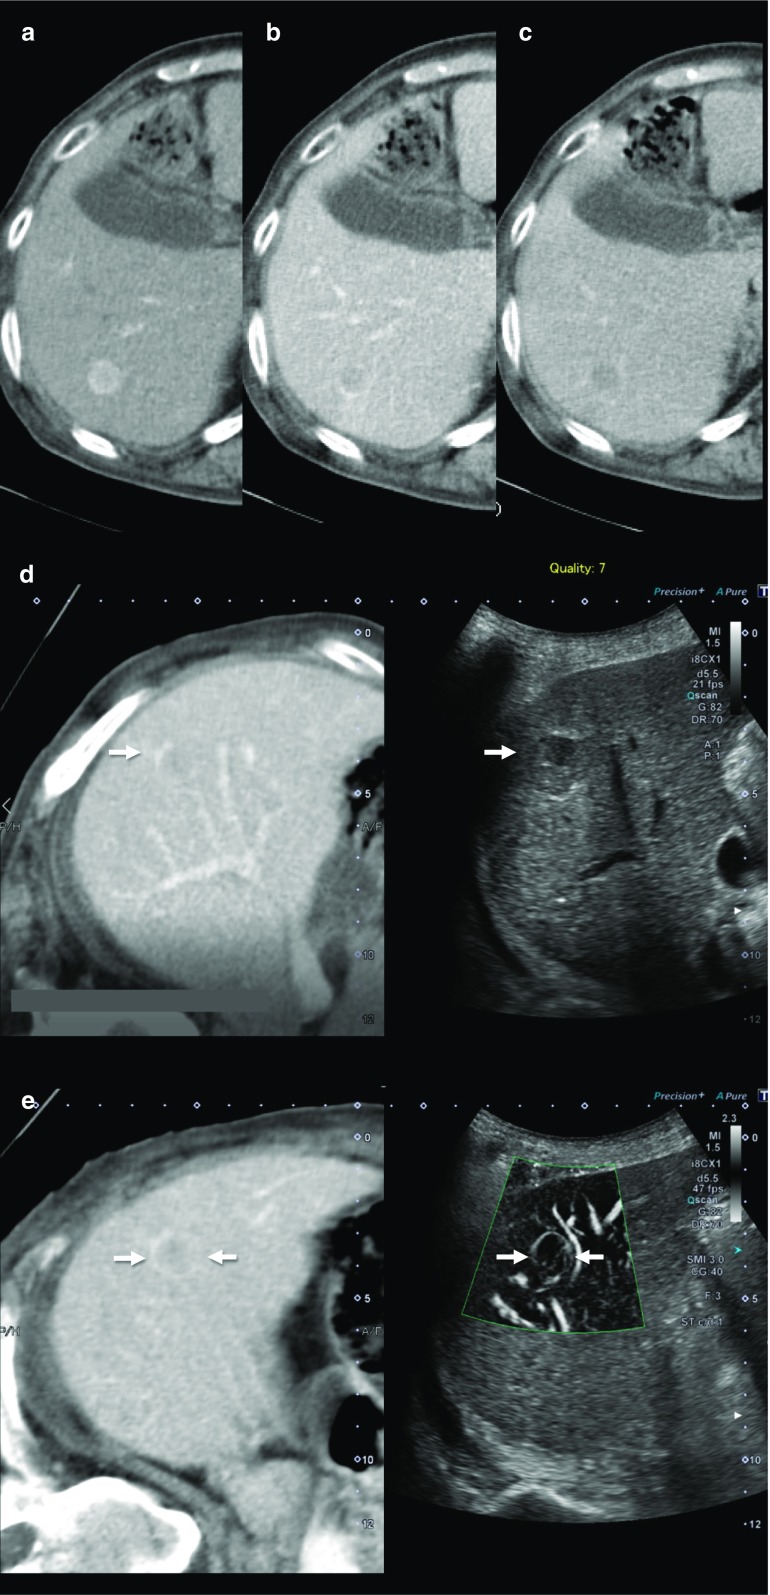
A case of recurrent HCC (maximum diameter 13 mm) in Segment 6. CT in the arterial phase shows hyper-enhancement (a). CT in the portal phase shows iso-enhancement (b). CT in the delayed phase shows hypo-enhancement (c). A hypoechoic tumor was found at the same site as the contrast-enhanced CT image in the portal phase using fusion imaging (d). Superb microvascular imaging (SMI) could produce very fine microvascular imaging with high resolution and a high frame rate (e)
